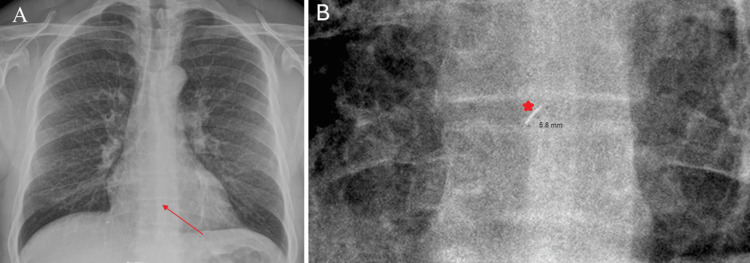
Initial radiological assessment. (A) Posteroanterior (PA) chest X-ray demonstrating a linear radiopaque foreign body (red arrow) superimposed on the lower cardiac silhouette and sternal region. (B) Magnified radiographic view providing a detailed visualization and measurement of the metallic density (red star).
